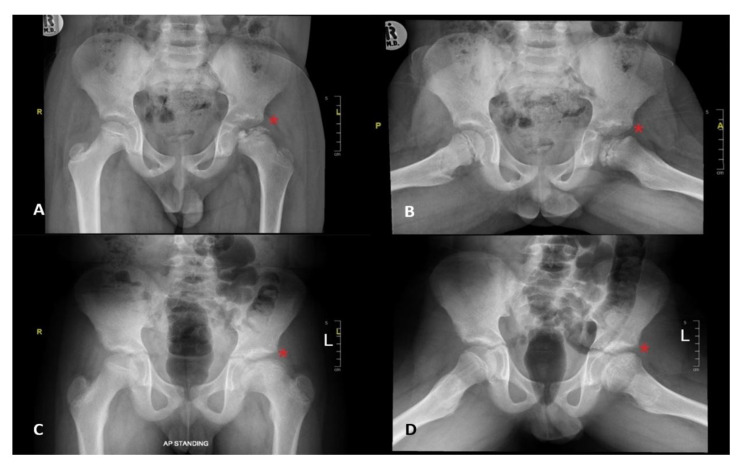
Example of pelvic x-rays of a patient with Legg-Calvé-Perthes disease aged younger than eight years before (A,B) and after (C,D) nonsurgical treatment.
